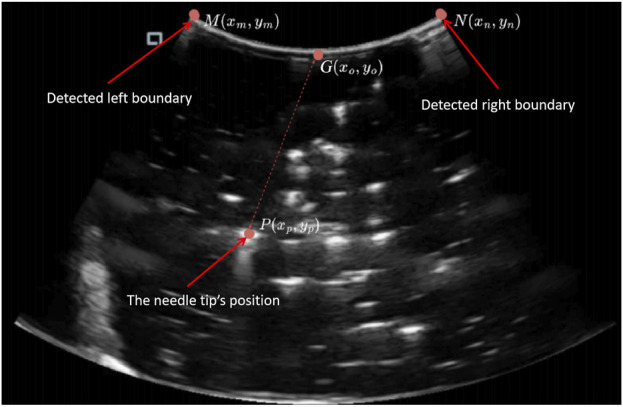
The coordinate transformation calculated in the ultrasound image.
